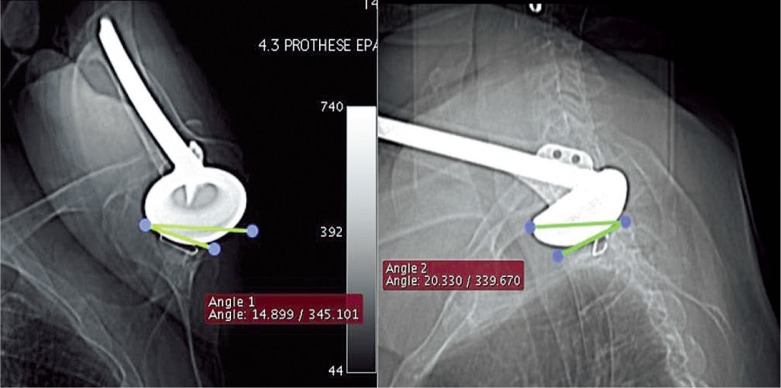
Coronal and sagittal scout views of the shoulder with the most severely limited active flexion angle of the series and also indicating the angle (non-ideal alignment) between the glenoid orientation and the CT-scan acquisition plane.
